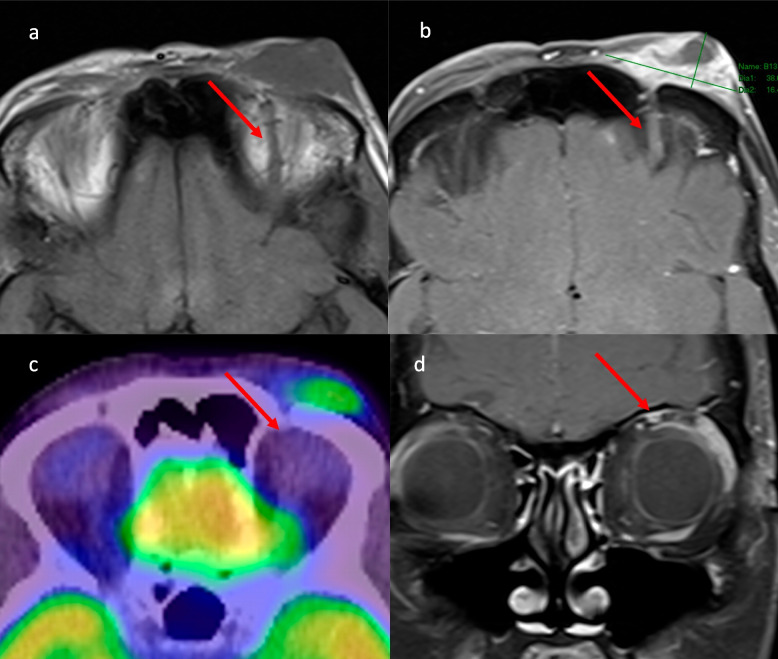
Perineural spread (PNS) in left V1, not detected by fluorodeoxyglucose-positron emission tomography computer tomography (FDG-PET/CT). Magnetic resonance imaging (MRI): (A) Axial T1, thickened left supraorbital nerve PNS. B MRI Axial T1 post-contrast fat saturation (FS), thickened left supraorbital nerve PNS with mild enhancement. C FDG-PET/CT axial image at the left superior orbital canal, no avidity in the PNS. PNS not detected on FDG-PET/CT. D MRI Coronal T1 FS post-contrast, demonstrates thickened enhancing left supraorbital nerve PNS
